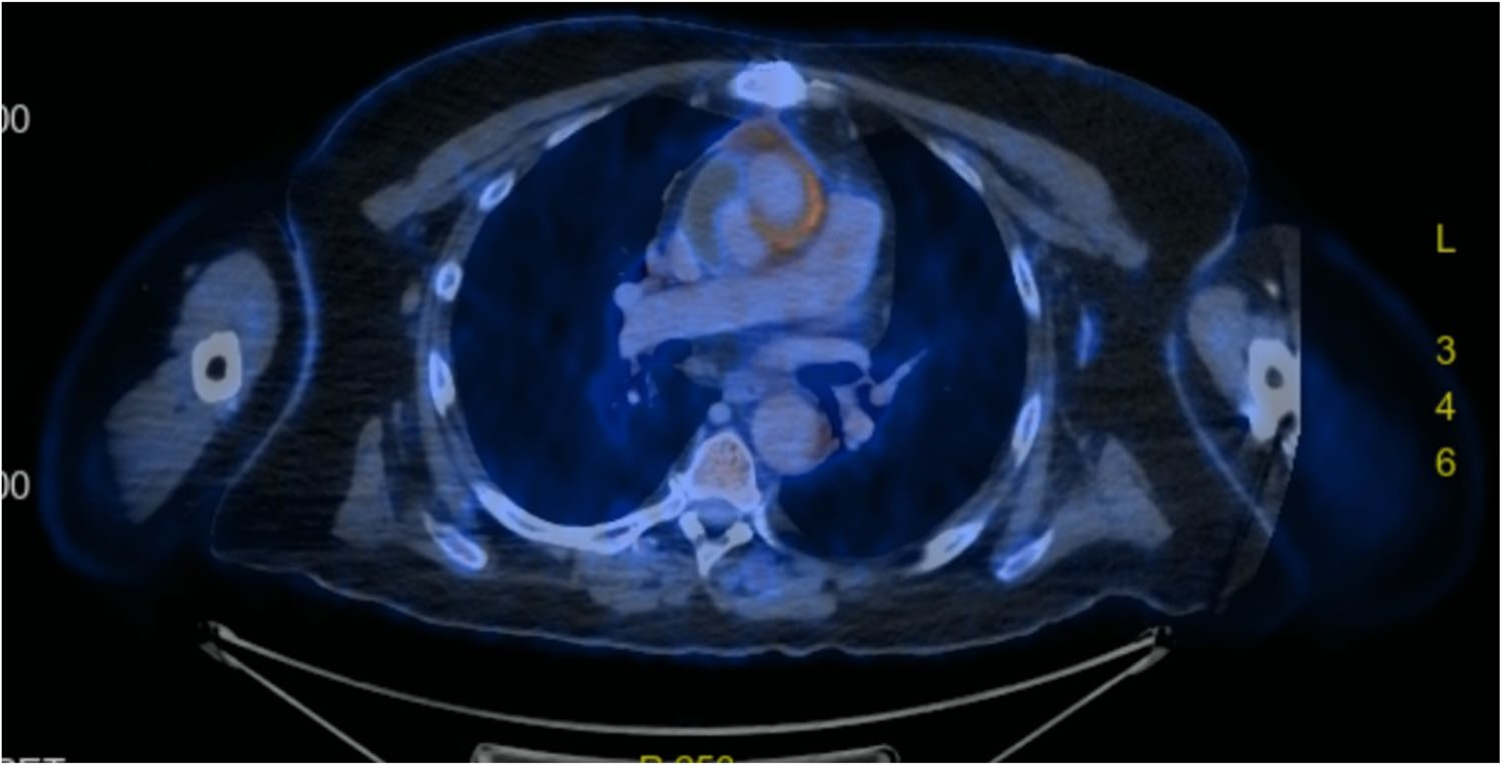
Preoperative positron emission tomography with 2-deoxy-2-[fluorine-18] fluoro-d-glucose (18F-FDG-PET/CT) showing uptake of an ascending aorta prosthetic graft highly suggestive of graft infection
